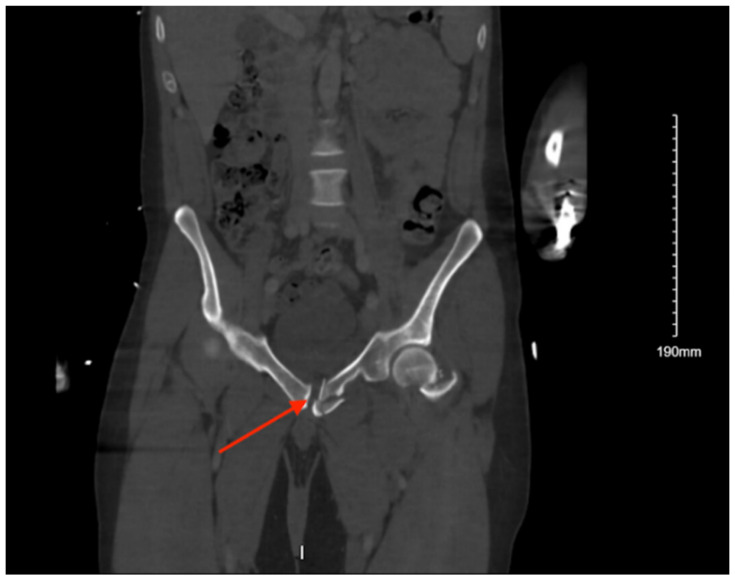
CT abdomen/pelvis images of the described fractures in case 11. Red arrows are used to indicate the location of pelvic fractures for easier identification.
